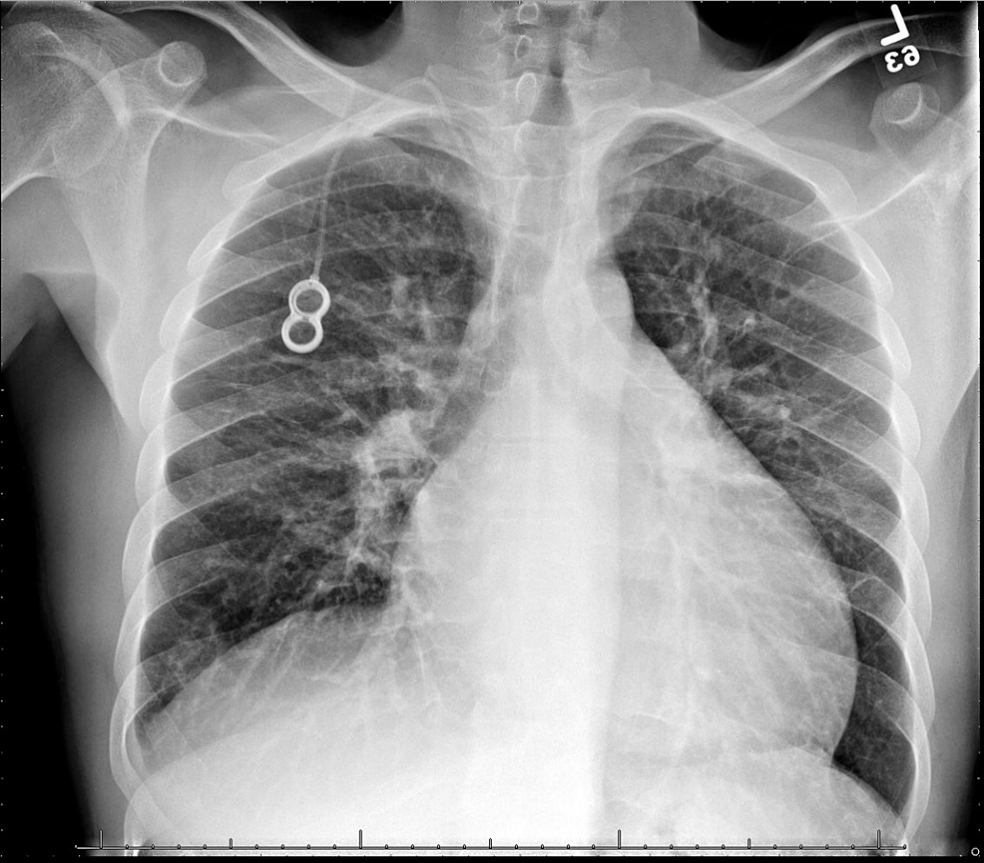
Chest x-ray on Admission Chest x-ray demonstrating cardiomegaly and interstitial pulmonary edema with cephalization.
